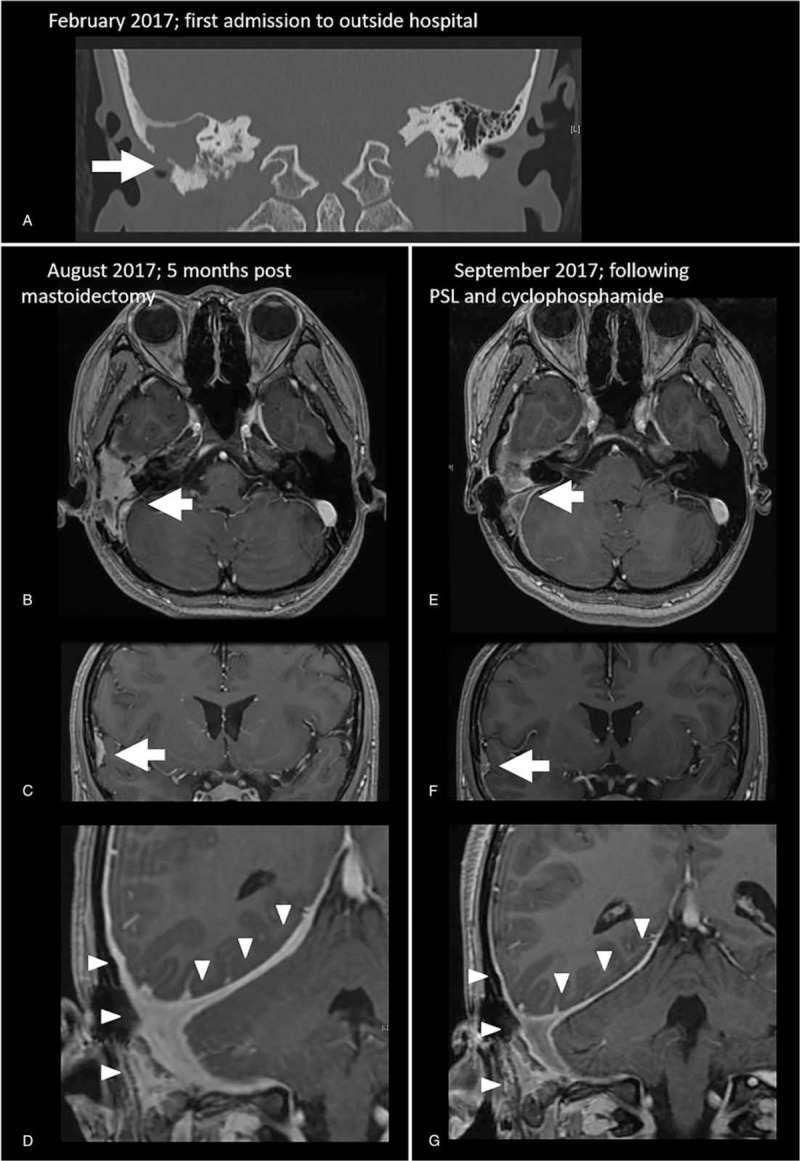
Image findings of mastoiditis and hypertrophic pachymeningitis. Computed tomography (CT) (A) and gadolinium-enhanced magnetic resonance imaging (MRI) (B) revealed right-sided mastoiditis (arrow). MRI revealed extensive hypertrophy of the right side dura mater (C, D) (arrow). MRI findings 1 month after the initiation of induction therapy revealed improvement of mastoiditis (E) and dura mater thickening (F, G).
